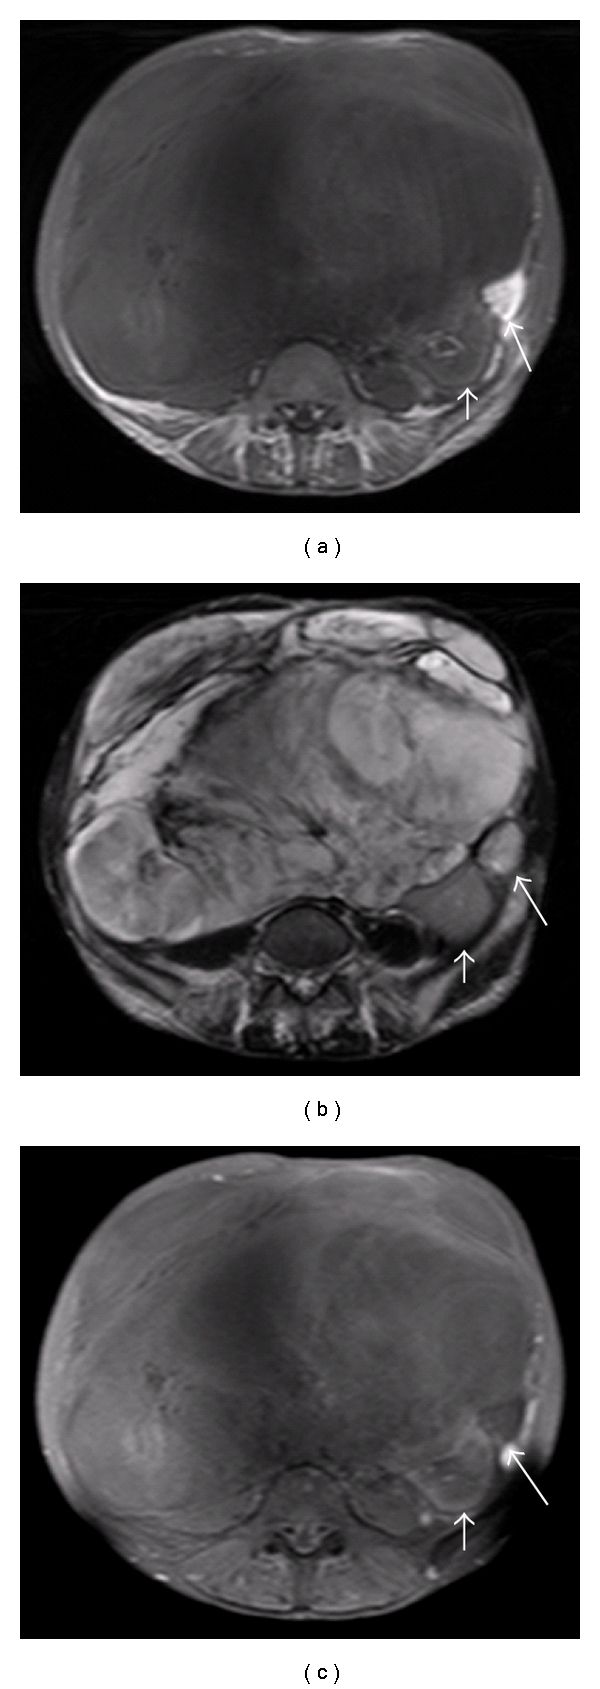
(a) shows T1-weighted images, (b) shows T2-weighted images, and (c) shows T1-weighted and fat suppression images on MRI. The giant tumor was T1 low intensity and T2 high intensity, but the small tumor was T1 high intensity, and with a changed low signal in fat suppression (long arrow). The right kidney was involved and pushed aside (small arrow).
